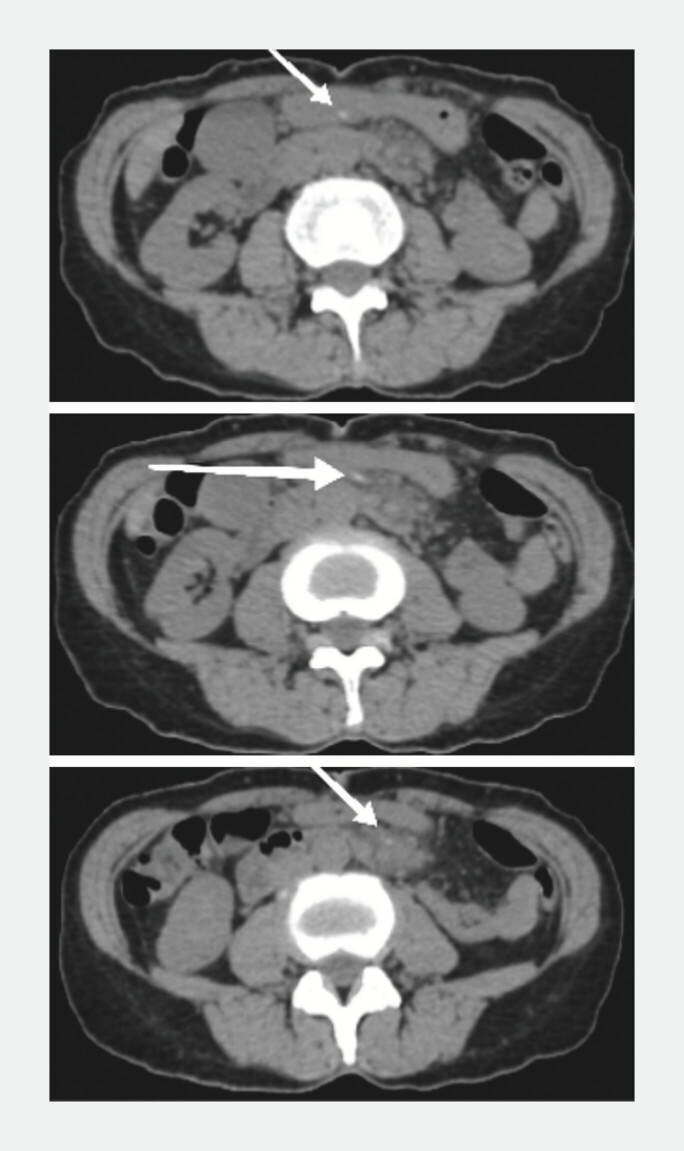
Abdominal computed tomography (CT) revealed a linear, slightly hyperdense structure in the gastric antrum region, extending outside the gastric lumen.
